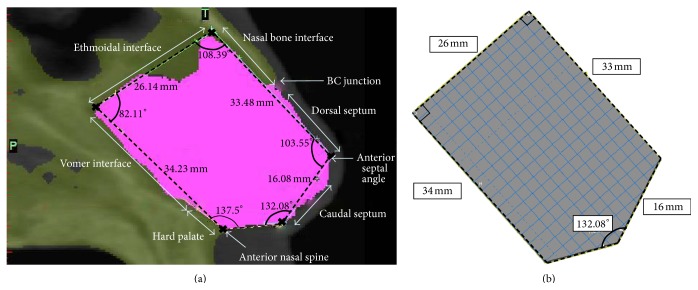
(a) Sagittal CT scan of the nasal septum with the indicated anatomical features and their measurements. (b) Idealized cartilaginous septum model created with the indicated measurements.
